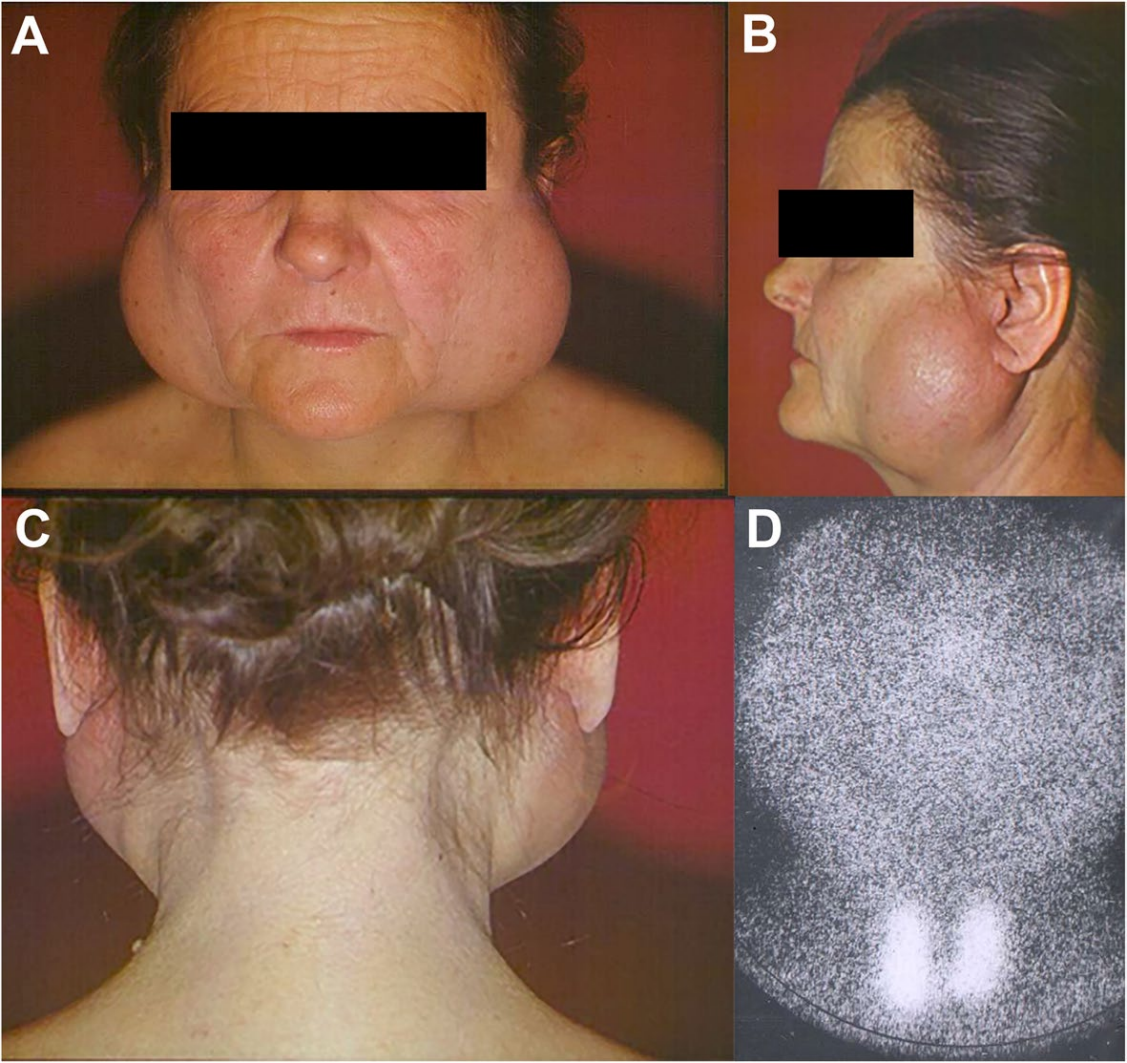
Presents the clinical features of a severe case of Sjögren’s syndrome with major lymphoid infiltration, without any remaining salivary gland tissue (A, B, and C). Salivary glands are not visible on the scintigraphy (D), only the thyroid gland
